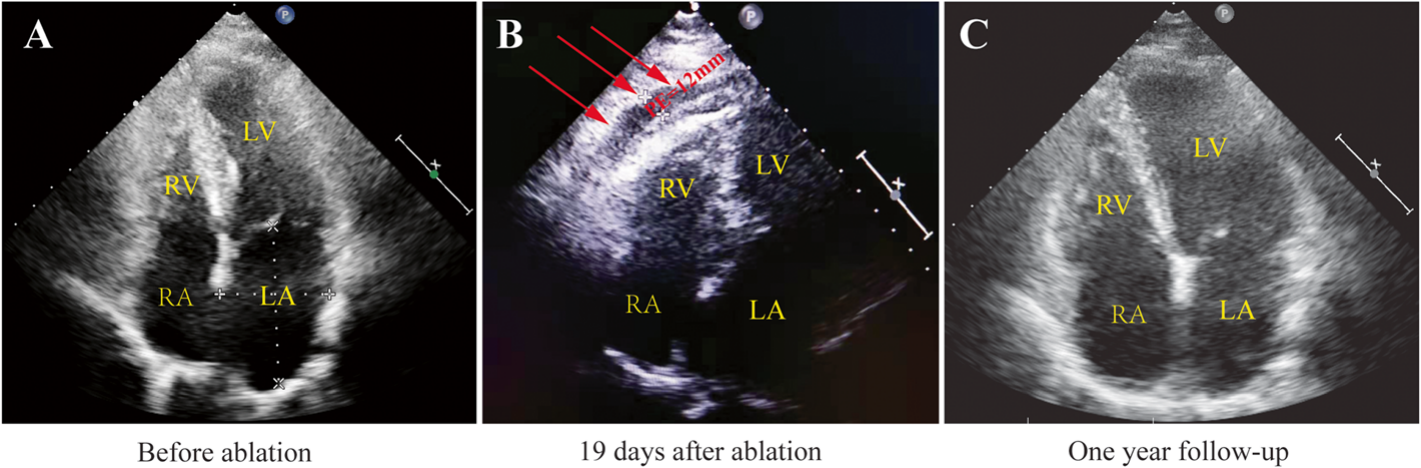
Echocardiographic images in 4-chamber view (a) before ablation, (b) at the time of cardiac tamponade, and (c) at 1-year follow-up are displayed
